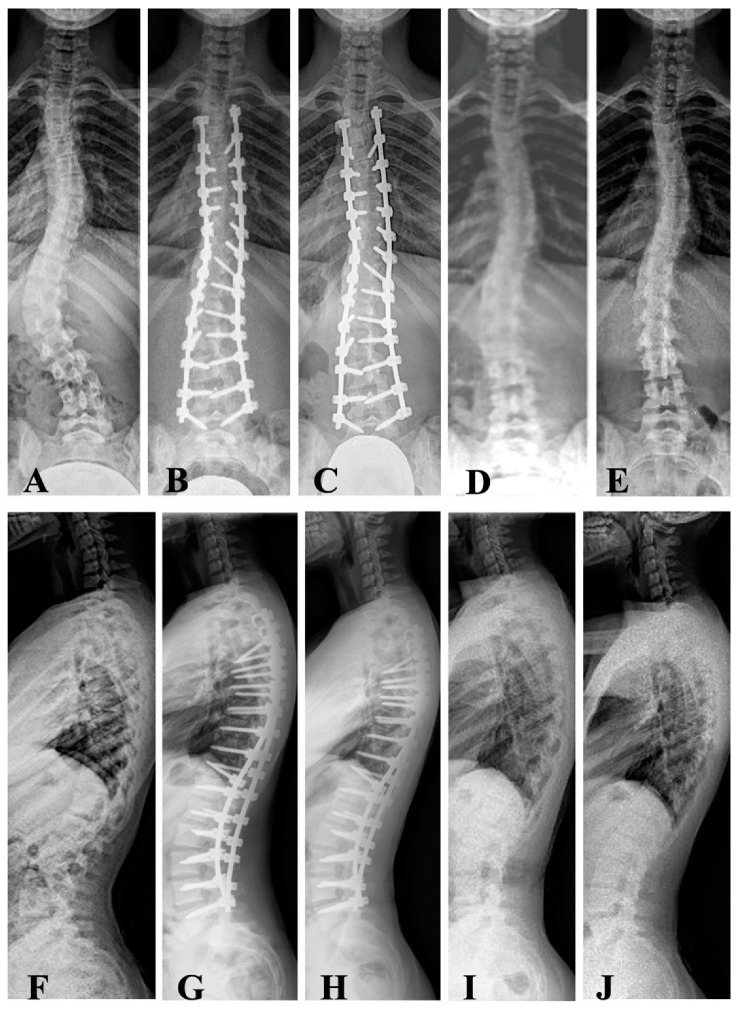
A 14-year-and-11-month-old girl with Lenke type 5 AIS (17/36/57), with T10-L2 kyphosis (+16°) and Risser 3 (A,F). Posterior pedicle instrumentation T4-L5 (B,G) was performed, achieving correction of the thoracolumbar and thoracic curve (B). In the sagittal plane, the correction of the thoracolumbar transit kyphosis (G) was also verified. The coronal and sagittal angular values remained unchanged until the removal of the vertebral instrumentation (C,H) and in the radiographic study after the removal of the implants (D,I). In the last radiographic control, two years after the removal of the instrumentation, the thoracic scoliosis (E) and the sagittal vertebral angular values remained unchanged (J).
